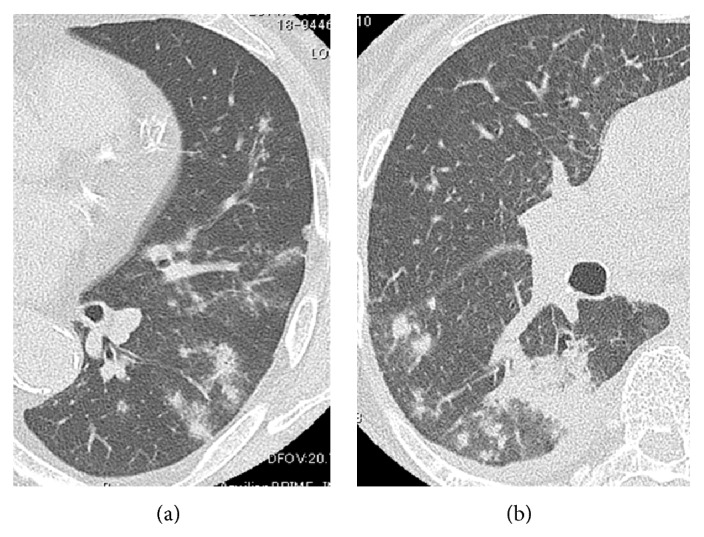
Representative chest CT images of pulmonary cryptococcosis in inclusive GGA or consolidation type. One patient showed multiple pale nodules with GGA in the left lung (a). Another patient showed multiple pale nodules, consolidation, and pleural effusion in the right lower lobe (b).
